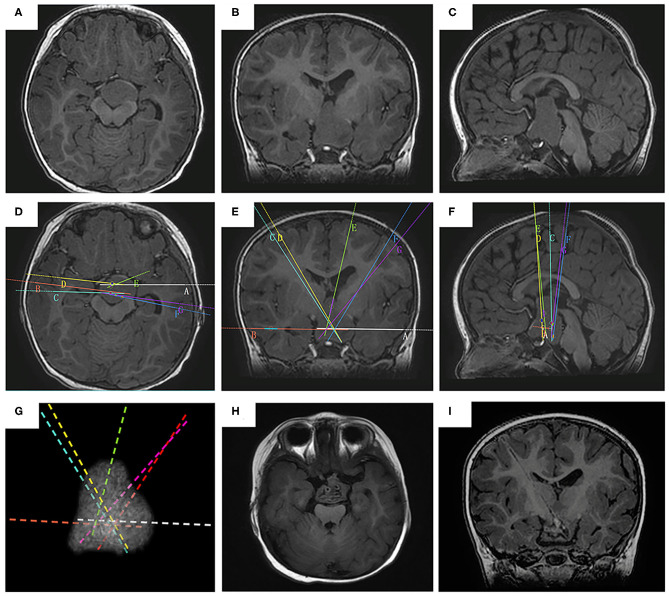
The SEEG-guided RF-TC in a giant type IV HH The preoperative MR images (A, axial image; B, coronal; C, sagittal) show a giant, bilaterally attached HH seat intra- and extra-third ventricle (case No. 2). The electrodes are designed to cover the whole interface (D, axial image; E, coronal; F, sagittal). (G) the 3D demonstration of the electrode trajectory. The postoperative MR images obtained 2 months after RF-TC (H, axial image; I, coronal) shows the coagulated lesion at the base of the hamartoma.
